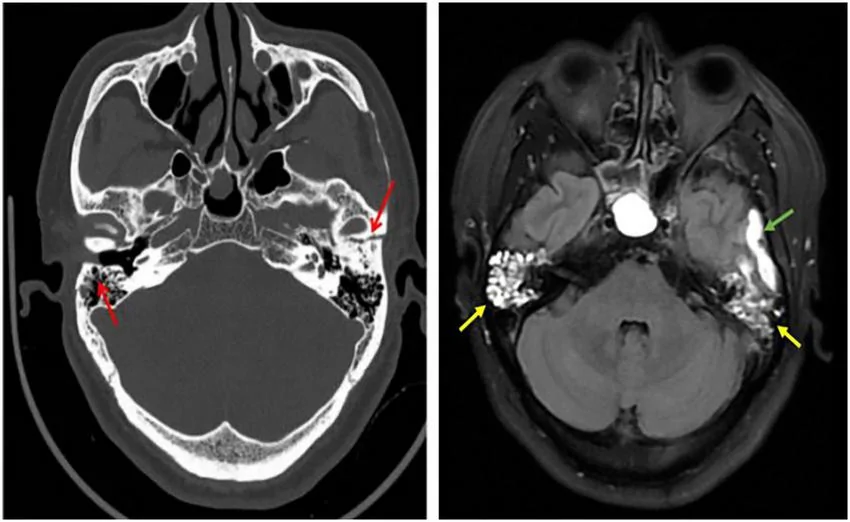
CT scan shows bilateral temporal bone fractures (indicated by the red arrow) and MRI reveals a left temporal lobe hematoma (indicated by the green arrow), fluid accumulation in bilateral mastoid processes and left sphenoid sinus (indicated by the yellow arrow).
